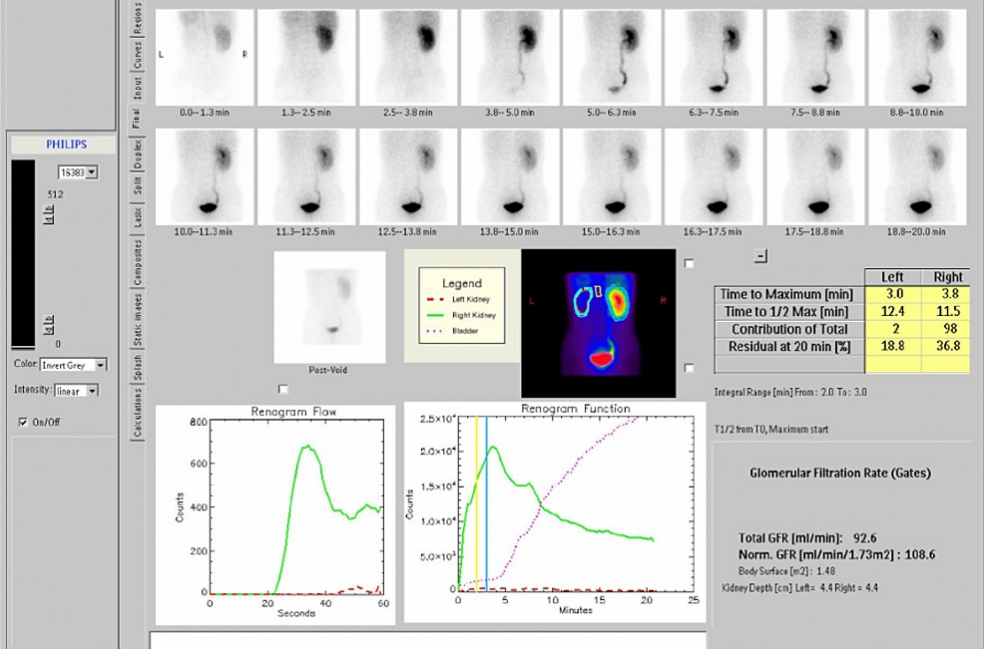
The Tc-99m DTPA renal dynamic scintigraphy showed complete loss of left kidney function. DTPA: diethylene-triamine-pentaacetate
